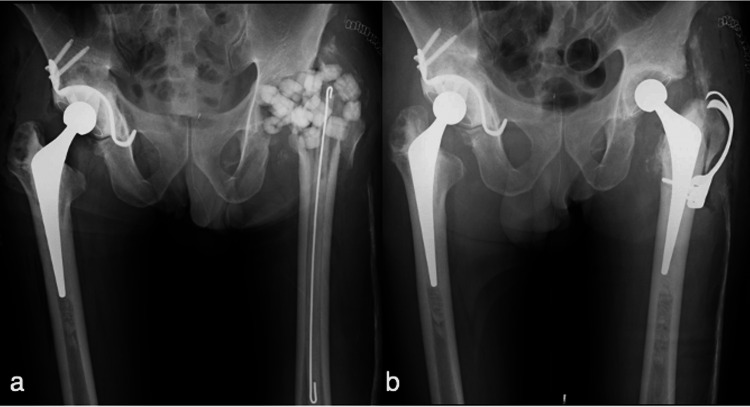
Anteroposterior radiograph. (a) Right-hip one-stage revision total hip arthroplasty (THA) was performed, and antibiotic-loaded acrylic cement beads and rod were deposited in the left hip after bilateral debridement. (b) Left-hip two-stage revision THA was performed 6.5 weeks later.
